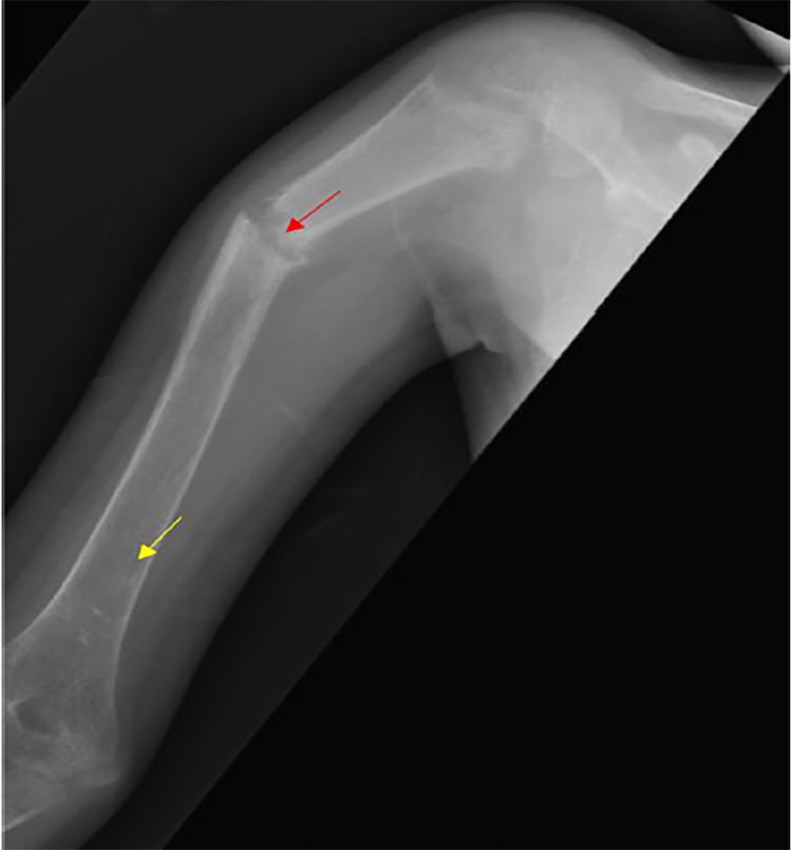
Radiograph of the right humerus of a nine-year-old female patient showing demineralization (yellow arrow) and healing fracture (red arrow).
